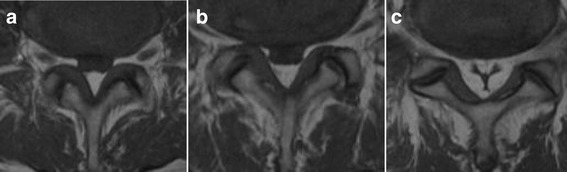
Lumbar axial magnetic resonance imaging. The adipose tissue compresses the thecal sac at L3–S1 levels, circumferentially. a L3-4 level, b L4-5 level, c L5-S1 level
